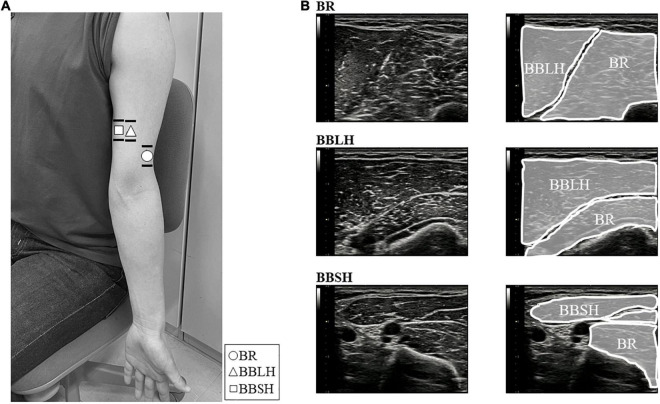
Images of the electrode locations and ultrasonogram for each muscle. (A) The location of surface (lines) and fine-wire (symbols) electrodes of the BR (○), BBLH (△), and BBSH (□). Surface electrodes were placed across the wire insertion of each muscle as shown in the horizontal lines. Fine-wire electrodes were inserted approximately where the symbols were shown. (B) The upper, middle, and lower panels represent the ultrasound images of the BR, BBLH, and BBSH muscles, respectively. BBLH, long head of the biceps brachii muscle; BBSH, short head of the biceps brachii muscle; BR, brachialis muscle.
